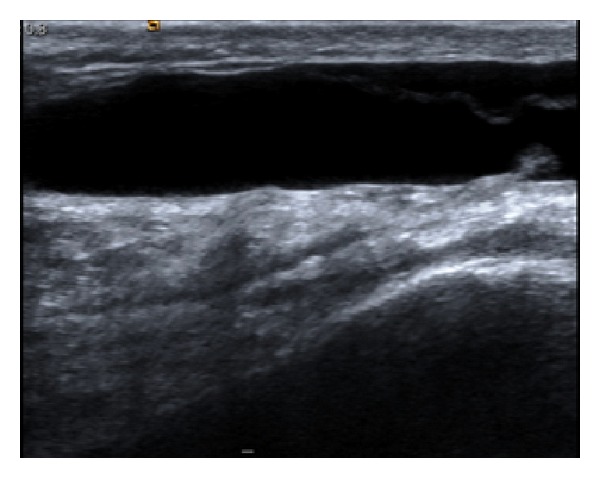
Ultrasound appearance of fluid collection lying anterior to extensor mechanism of right knee.
